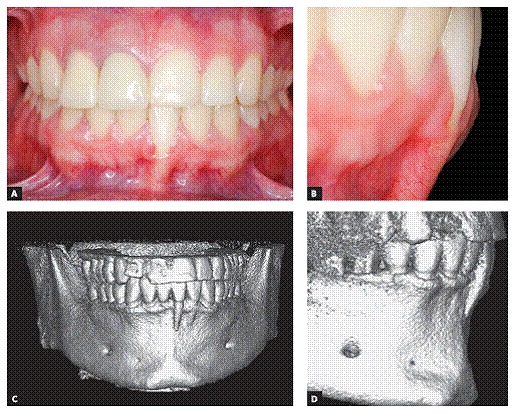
CT scan revealing absence of apical as well as buccal bone plate of a mandibular incisor with gingival recession. Note the presence of inflammation associated with dental plaque and occlusal interference.
